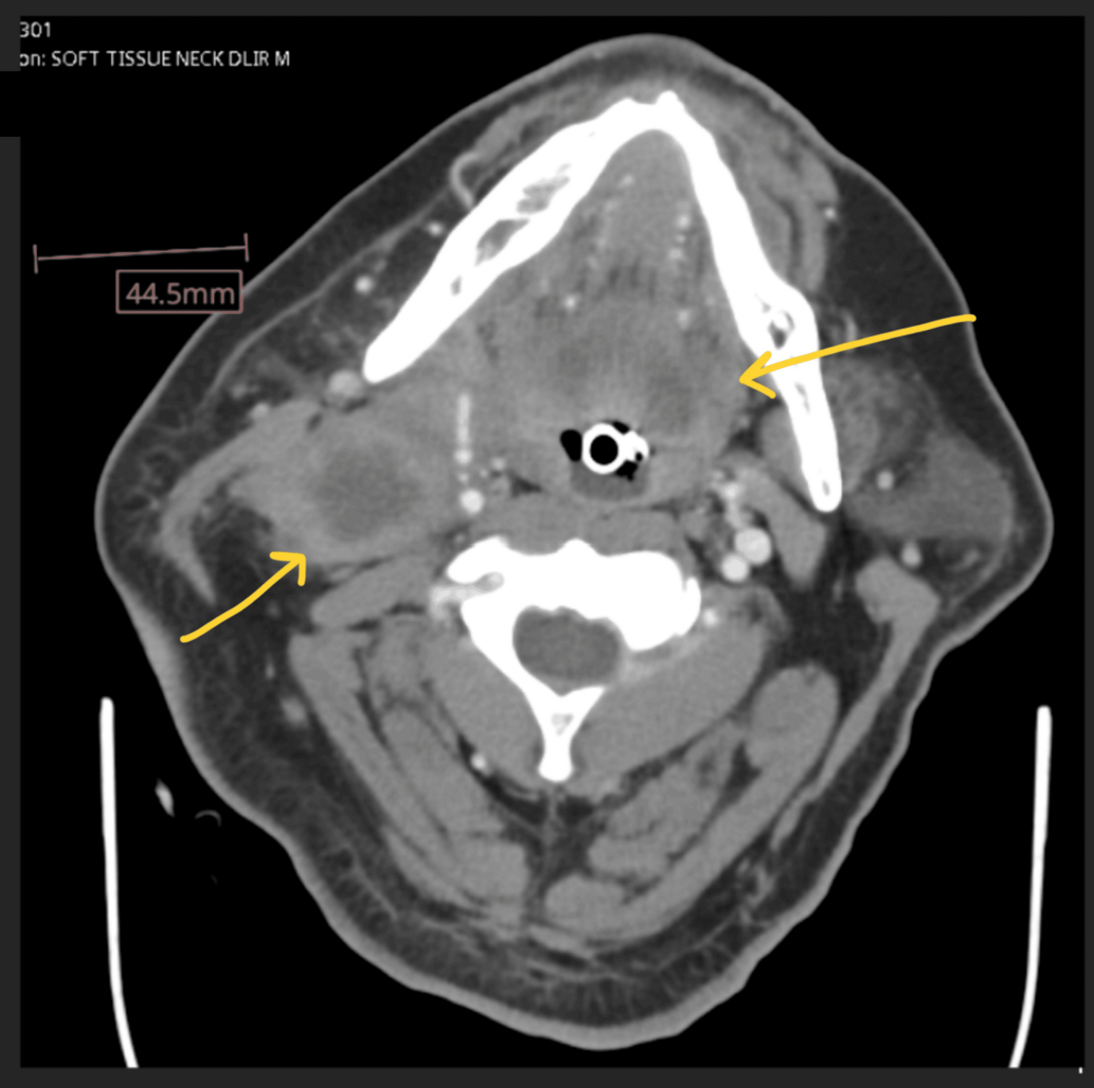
Axial view of CT soft tissue neck with contrast
